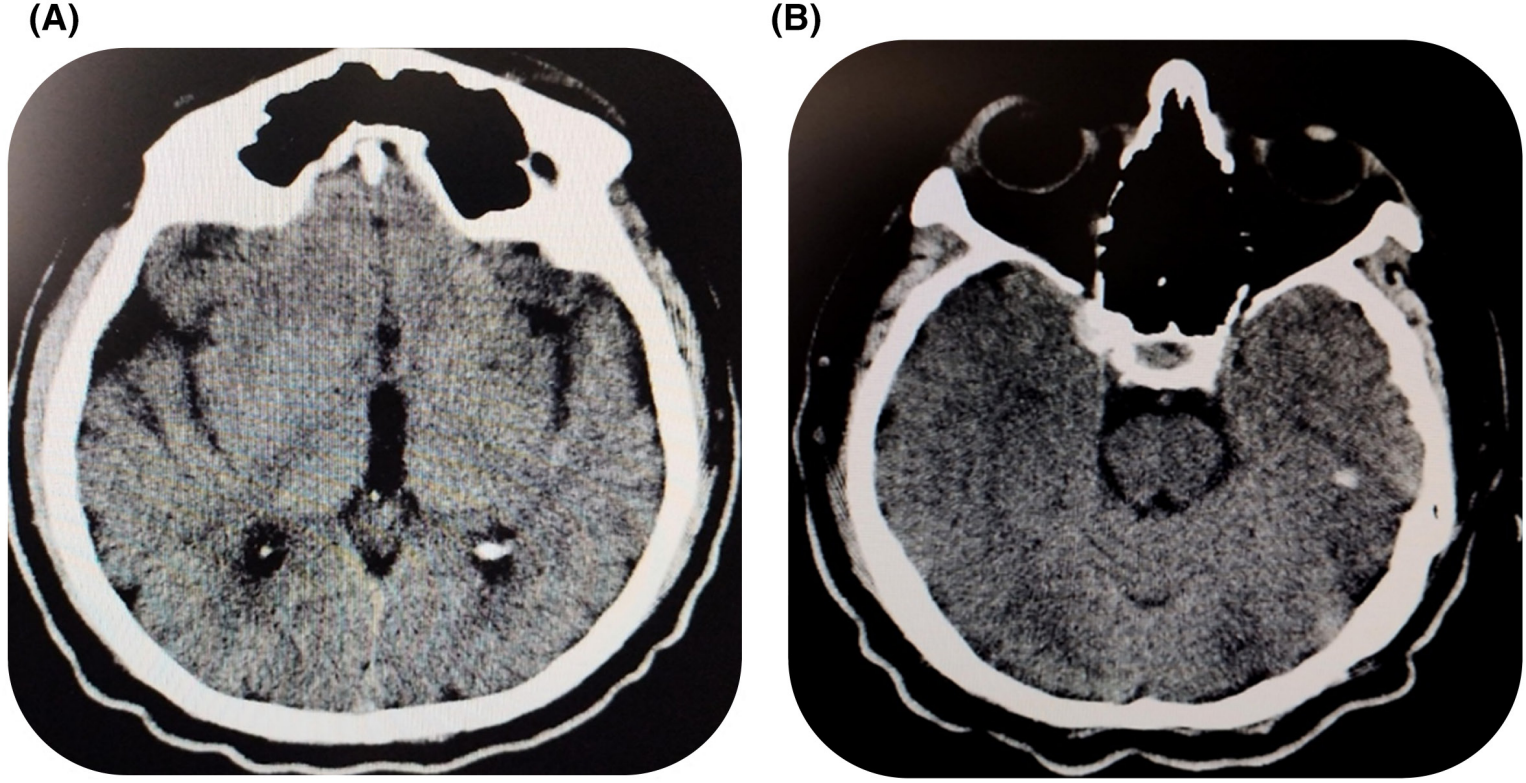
Brain CT (A, B) Multiple hypo attenuating foci are present beneath the frontal cortical subcortical regions and around the lateral ventricles on both sides. There are also multiple cystic lesions in the basal ganglia on both sides.
